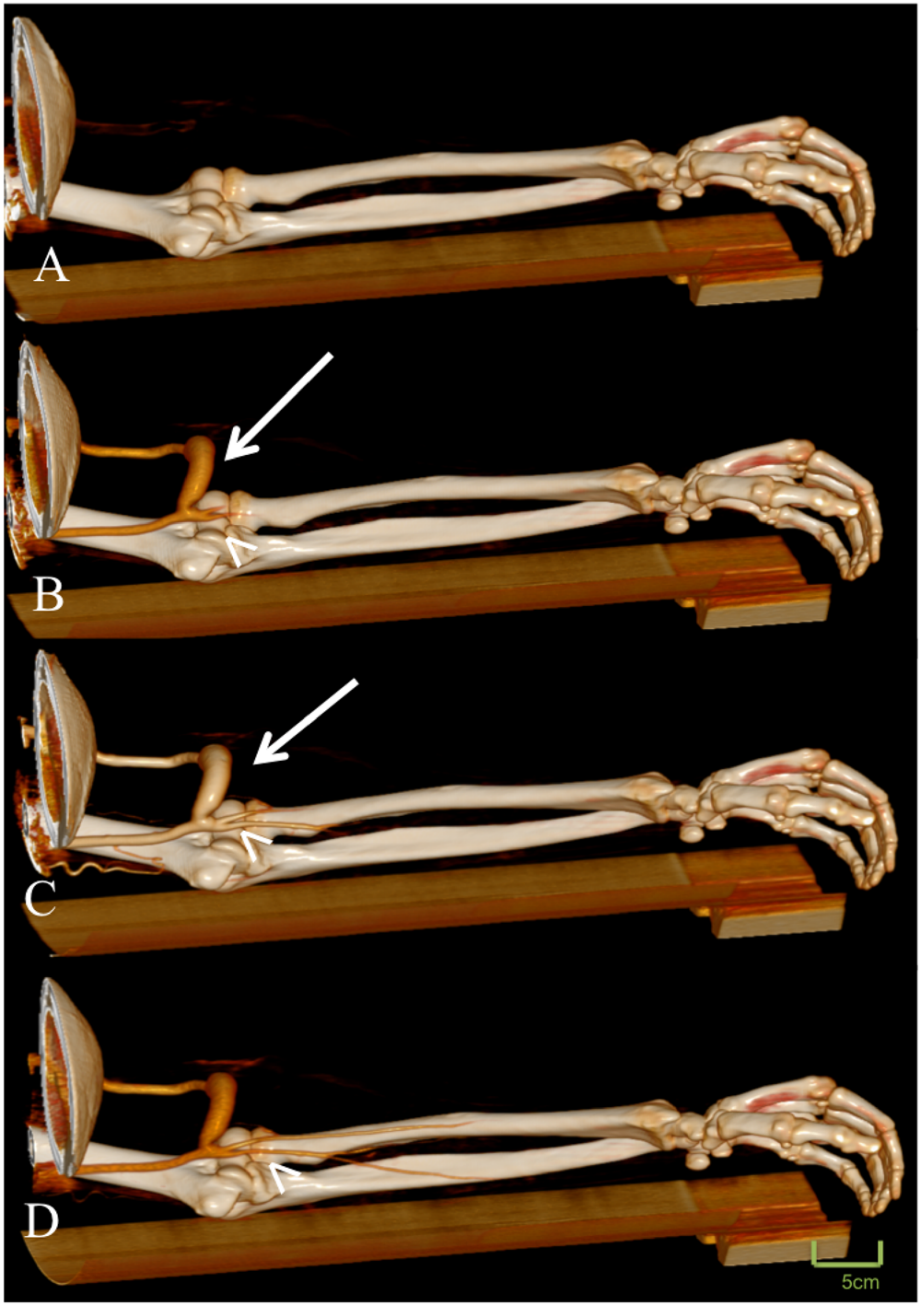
Displayed is a 48-year old male patient with new onset of arm pain. The patient had revision surgery due to a failing arterio-venous failure two weeks prior to the examination. Time-resolved dynamic CT angiography displayed an early filling of the efferent vein and a delayed filling of the brachial artery (arrow head) indicating a high-flow shunt (see also supplementary video 1).
